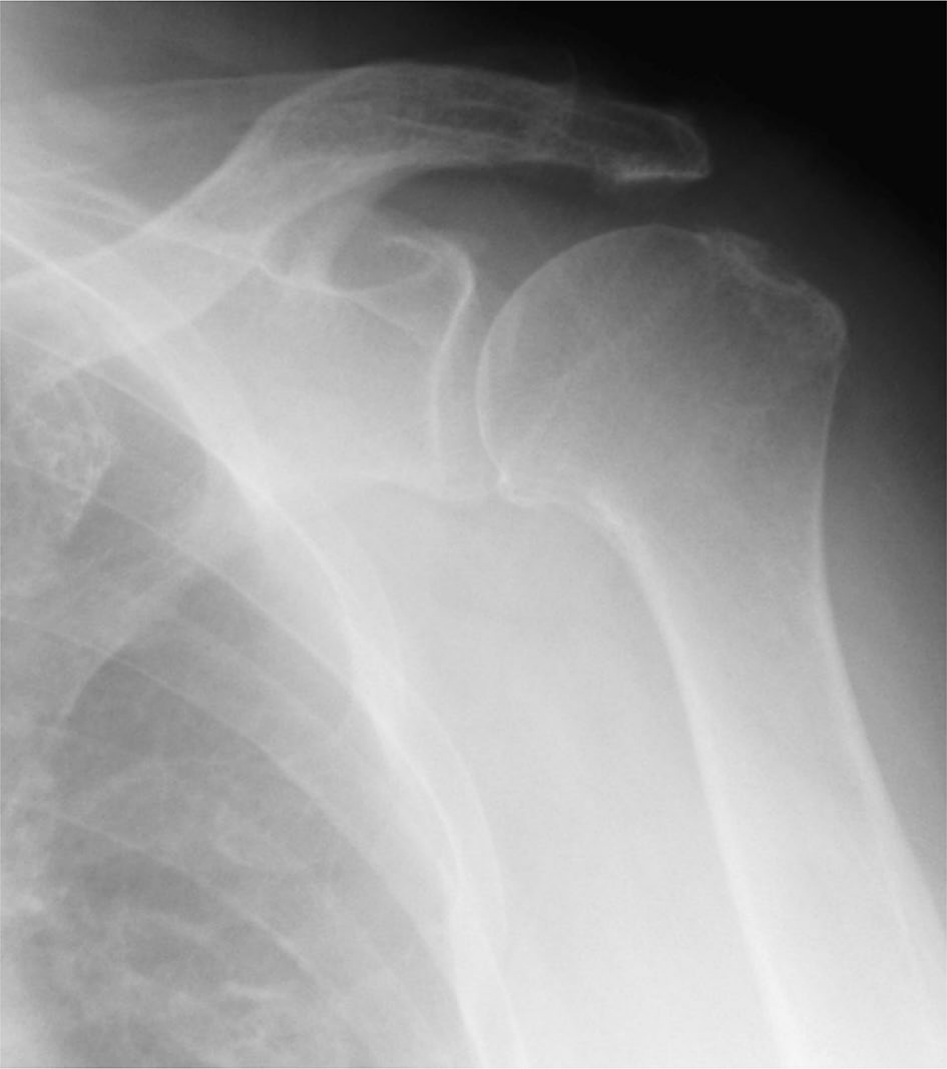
The figure shows the anteroposterior radiograph of Samilson-Prieto grade 2.
